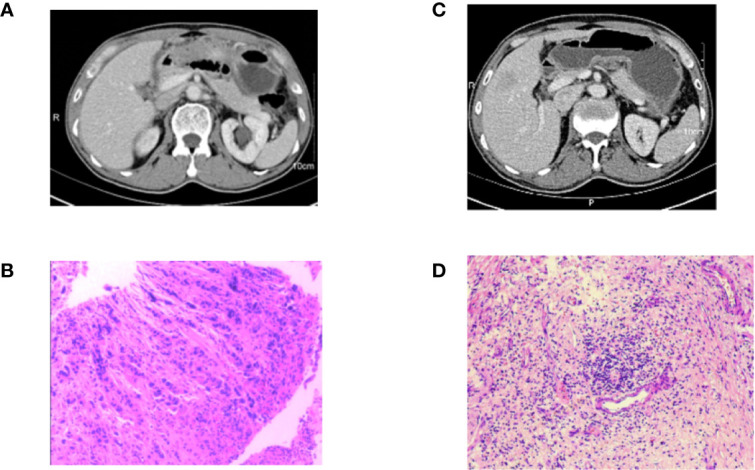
Pathology and imaging data of patient 3. (A) The CT of the patient at the first examination; (B) The gastroscopic pathology that adenocarcinoma of the lesser curvature of the gastric body; (C) Enhanced CT scan was performed, suggesting that the tumor was further smaller than first examination; (D) Postoperative pathology of the patient.
